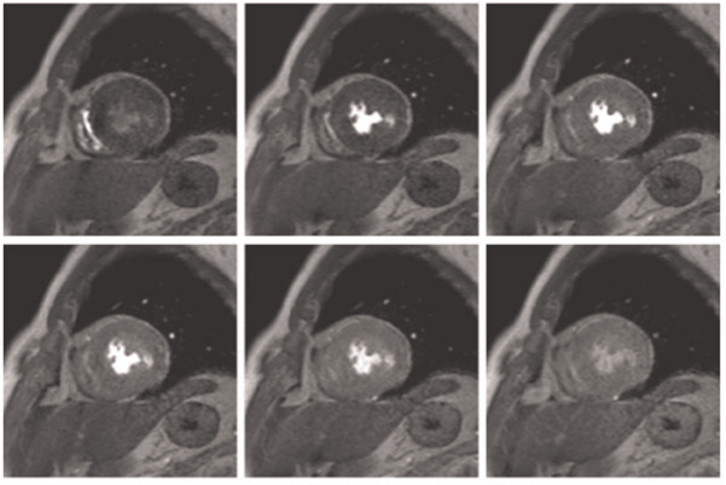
Rest perfusion MRI acquired with 3 T MR imager, 32 channel cardiac coils and k-t SENSE in a patient with triple vessel disease. Rest perfusion MRI is normal and no endocardial banding artifact is observed.
